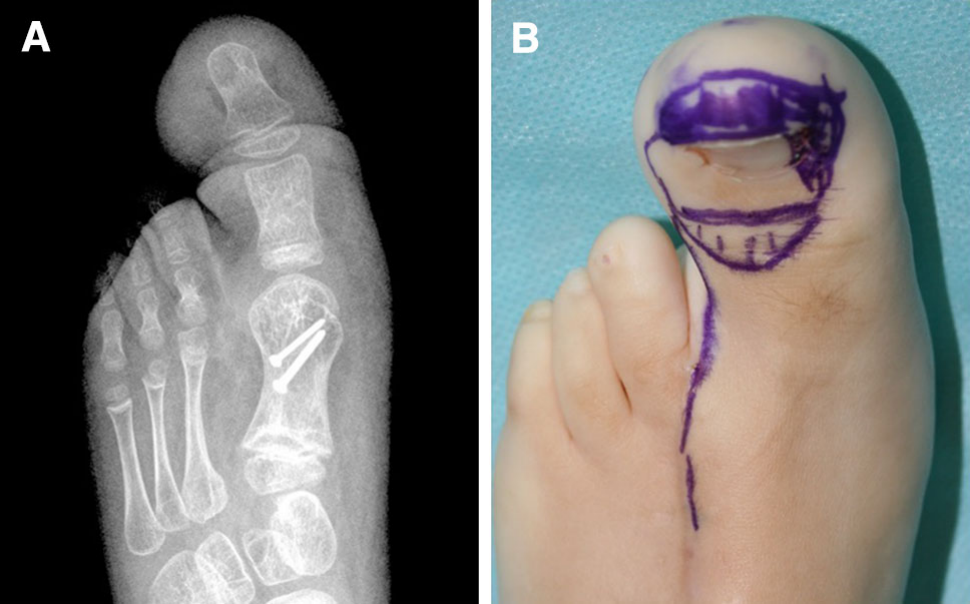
Case 1: Preoperative radiographs (a) and planned incisions (b). Note the hatched area where the flap was to be transferred
